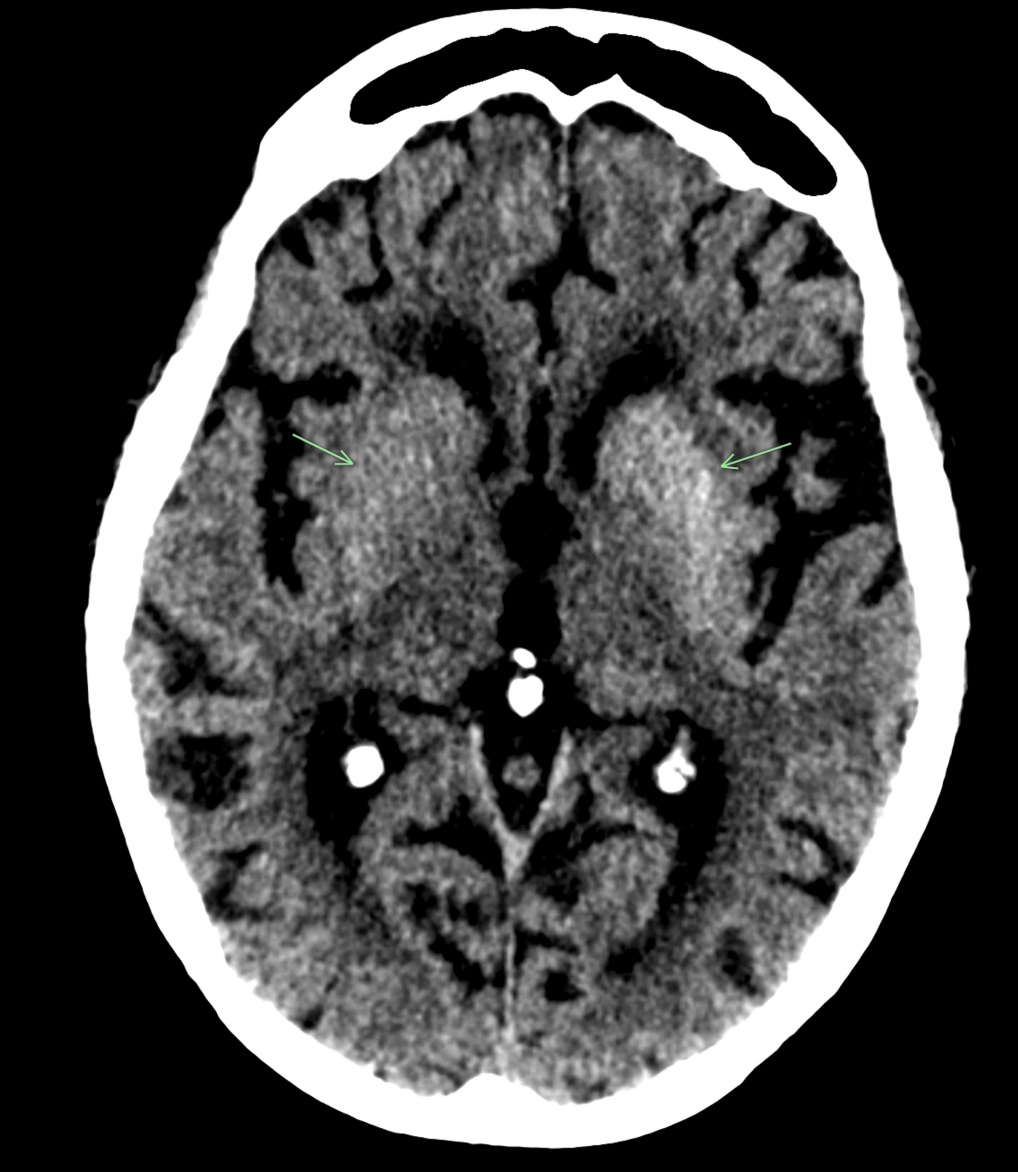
CT head showing hyperdensity in the region of the left basal ganglia Bilateral basal ganglia are shown by the green arrows. An obvious hyperdensity is noted on the left side, as pointed by the arrow. No surrounding oedema or mass effect is present.
